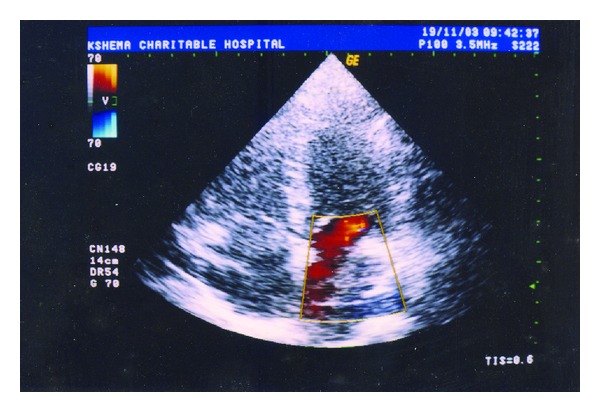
Color Doppler ultrasonographic image showing the regurgitation of blood into the left atrium.
